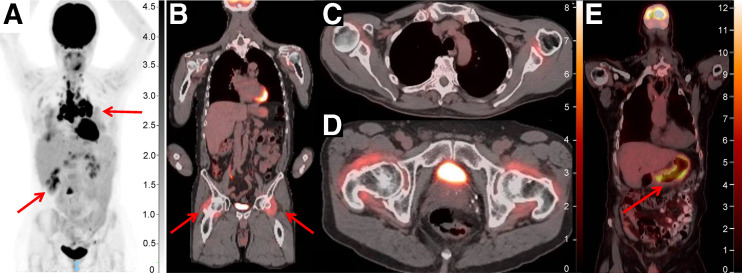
PET/CT for detection of immune-related adverse events. (A) PET/CT displaying hypermetabolic pulmonary nodules, subcarinal and hilar adenopathy, and hypermetabolic liver lesions (arrows), initially concerning for metastatic disease but later biopsied and found to be consistent with ICI-related sarcoidosis. (B) Increased ^18^F-FDG uptake bilaterally in hips (arrows) and shoulders consistent with immune-mediated arthritis. (C and D) Two axial views of ^18^F-FDG uptake in shoulders and hips. (E) Diffuse ^18^F-FDG uptake within posterior and posterior-lateral gastric wall consistent with immune-mediated gastritis, noted before onset of symptoms. Unit of measure for intensity bar is SUV = C(T)/[injection dose (MBq)/patient weight (kg)]).
